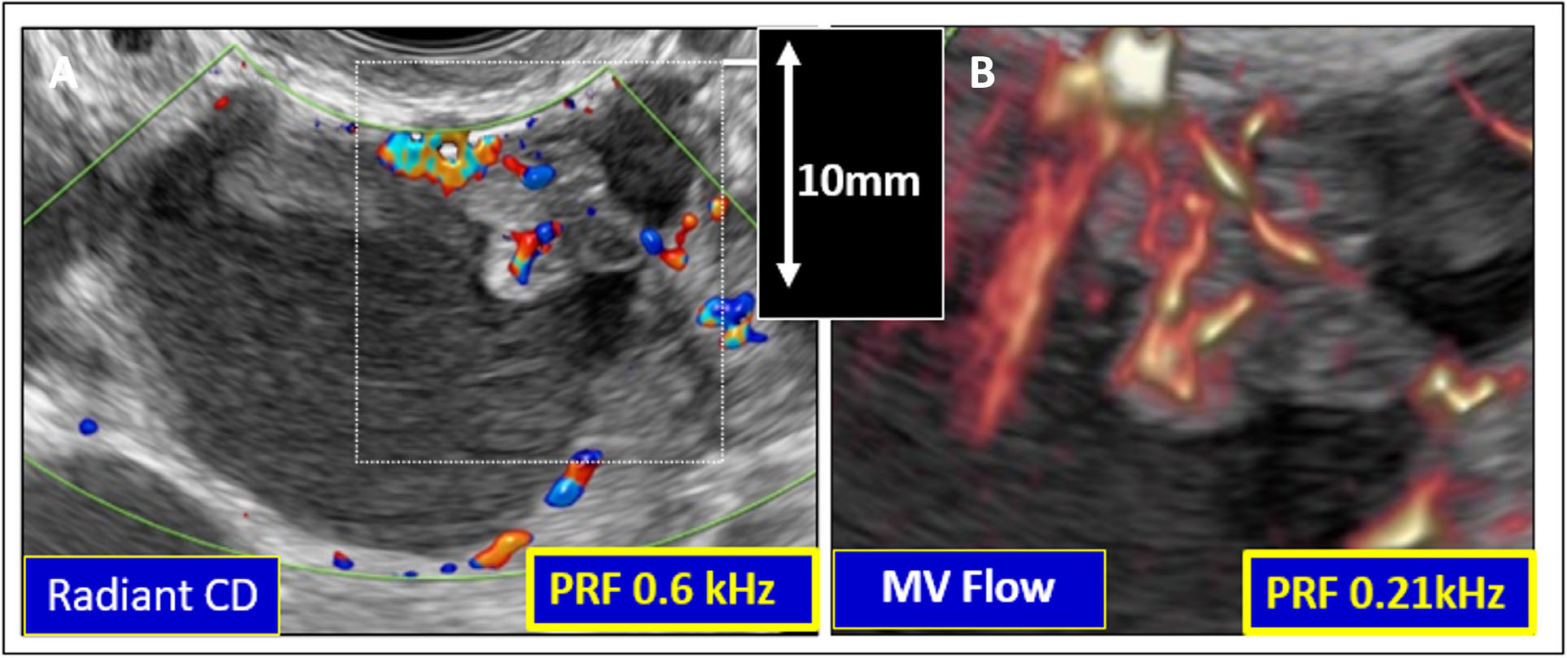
Ultrasound imaging of mucinous borderline ovarian tumor by radiant color Doppler US (CDUS) using a PRF of 0.6 kHz and microvascular imaging (MVI) using a PRF of 0.21 kHz. Note the difference in the details of the vascular patterns.
